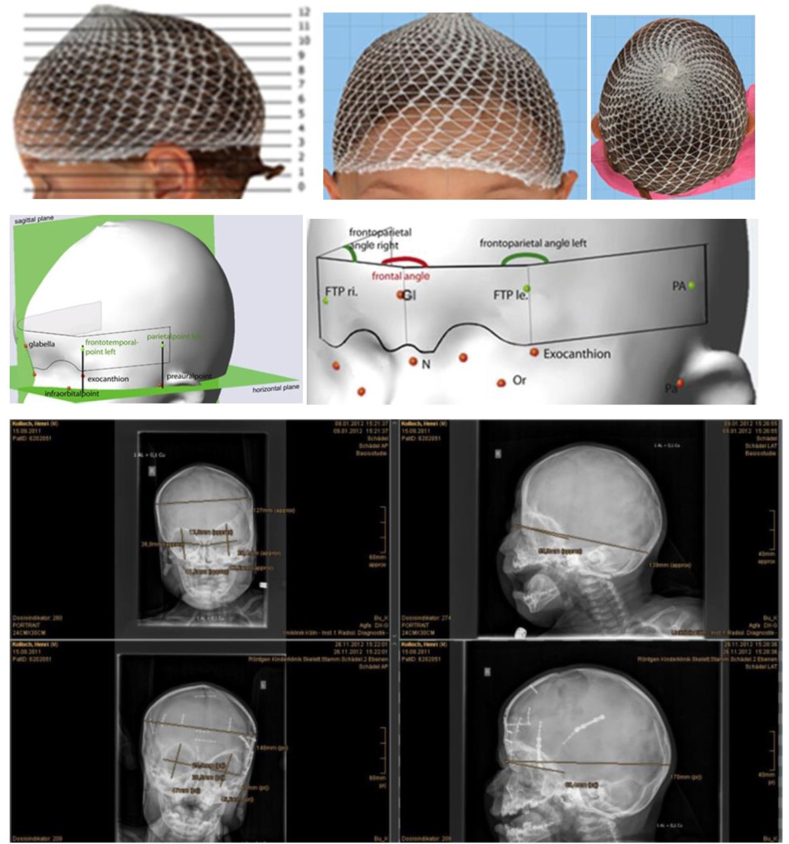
a-i) 3-D photometry with soft tissue points and pre- and postoperative X-ray image of a patient with subtle trigonocephalus; a) View of the head with mesh cap for smoothing the scan surface from the lateral left, subdivision of the head into 12 layers; b) View of the head with mesh cap for smoothing the scan surface from the front; c) top view of the head with mesh hood for smoothing the scan surface; d) 3-D photometry with soft tissue points in the view from the lateral left with sagittal and horizontal plane (green), glabella (red), infraorbital point, frontotemporal point (green), exocanthion, parietal point (green) and preaural point marked; e) 3-D photometry with soft tissue points and angles in the view from the frontal left, FTP ri and le: frontotemporal point right and left (green); Gl: glabella (red); N: nasion (red); Or: infraorbital point (red); Pa: preaural point (red); PA: parietal point (green); frontal angle (red); frontoparietal angle right and left (green). The frontal angle was measured between the nasion and the lateral edges of the orbit. The frontoparietal angles were measured on the right and left between the lateral edge of the orbit, the nasion and a fixed parietal point, approx. 1 cm above the base of the ear. f) Preoperative X-ray image in the frontal view (p.a.) with measurement points marked (maximum skull width, interorbital distance, orbital height/width). g) Preoperative X-ray image in the lateral view with marked measuring points (maximum skull length (nasion-inion), length of the anterior cranial fossa (sella-nasion)). h) Post-operative X-ray image in frontal view (p.a.) with osteosynthesis material in place after frontoorbital advancement (FOA) and measurement points marked (maximum skull width, interorbital distance, orbital height/width). i) postoperative X-ray image in the lateral view with osteosynthesis material in place after frontoorbital advancement (FOA) and measurement points marked (maximum skull length (nasion-inion), length of the anterior cranial fossa (sella-nasion)). (For interpretation of the references to colour in this figure legend, the reader is referred to the Web version of this article.)
